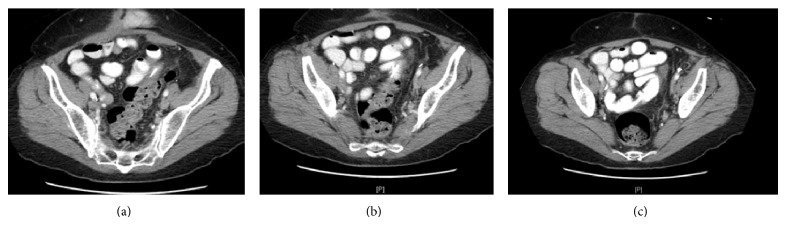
Serial images from a CT of the abdomen and pelvis with IV and oral contrast show the peritoneal catheter in the lower pelvis. The catheter approaches and then enters a loop of small bowel, confirming the position of the catheter as seen in the prior fluoroscopic images.
